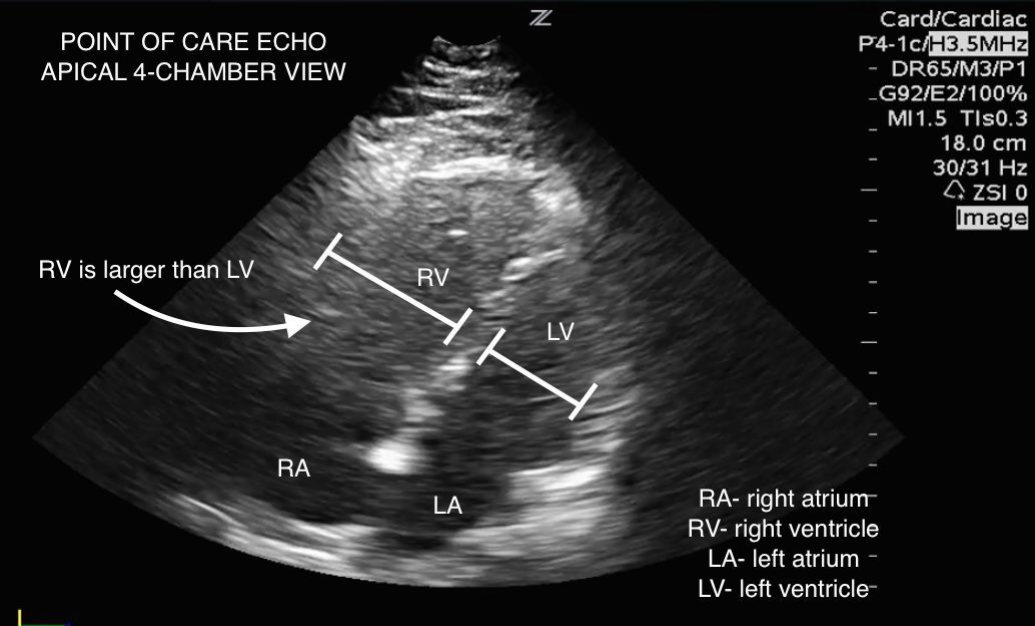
Point-of-care echocardiogram in apical 4-chamber view demonstrates significant right ventricle dilation.
